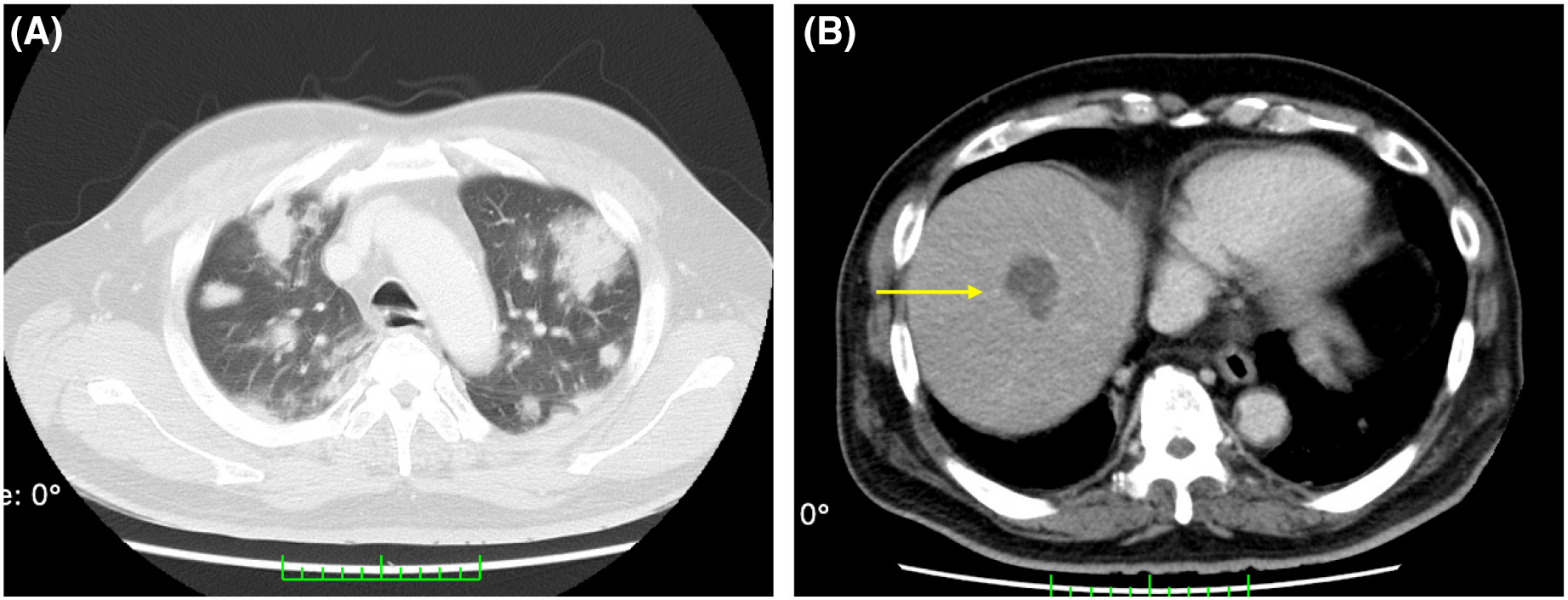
CT scan without contrast‐enhancement on the 3rd hospital day showing cotton‐like shadow and rounded infiltrate with air bronchogram; the margin of the infiltrate is fluffy, and the inside is granular in the lung (A); the liver abscess is enlarged to 33 mm on CT scan with contrast‐enhancement (B).
